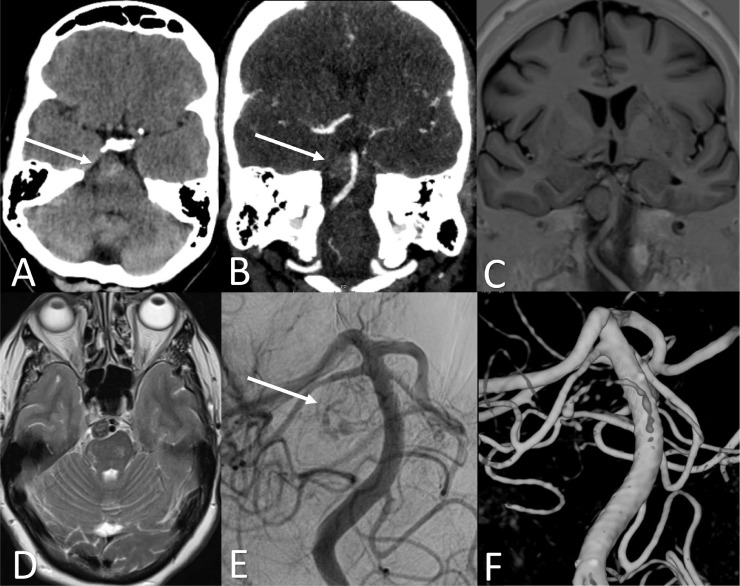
Different imaging modalities showing the basilar artery perforator aneurysm. (A) A prepontine mass on CT after 2 weeks of presentation; (B) CT with contrast did not show enhancement; (C) on T1 sequence MRI the aneurysm appeared iso-intens; (D) on T2 sequence MRI the aneurysm showed mixed intensities but mainly hypo-intens; (E) angiography showed a partially thrombosed aneurysm; (D) 3D reconstruction of the aneurysm on angiography.
